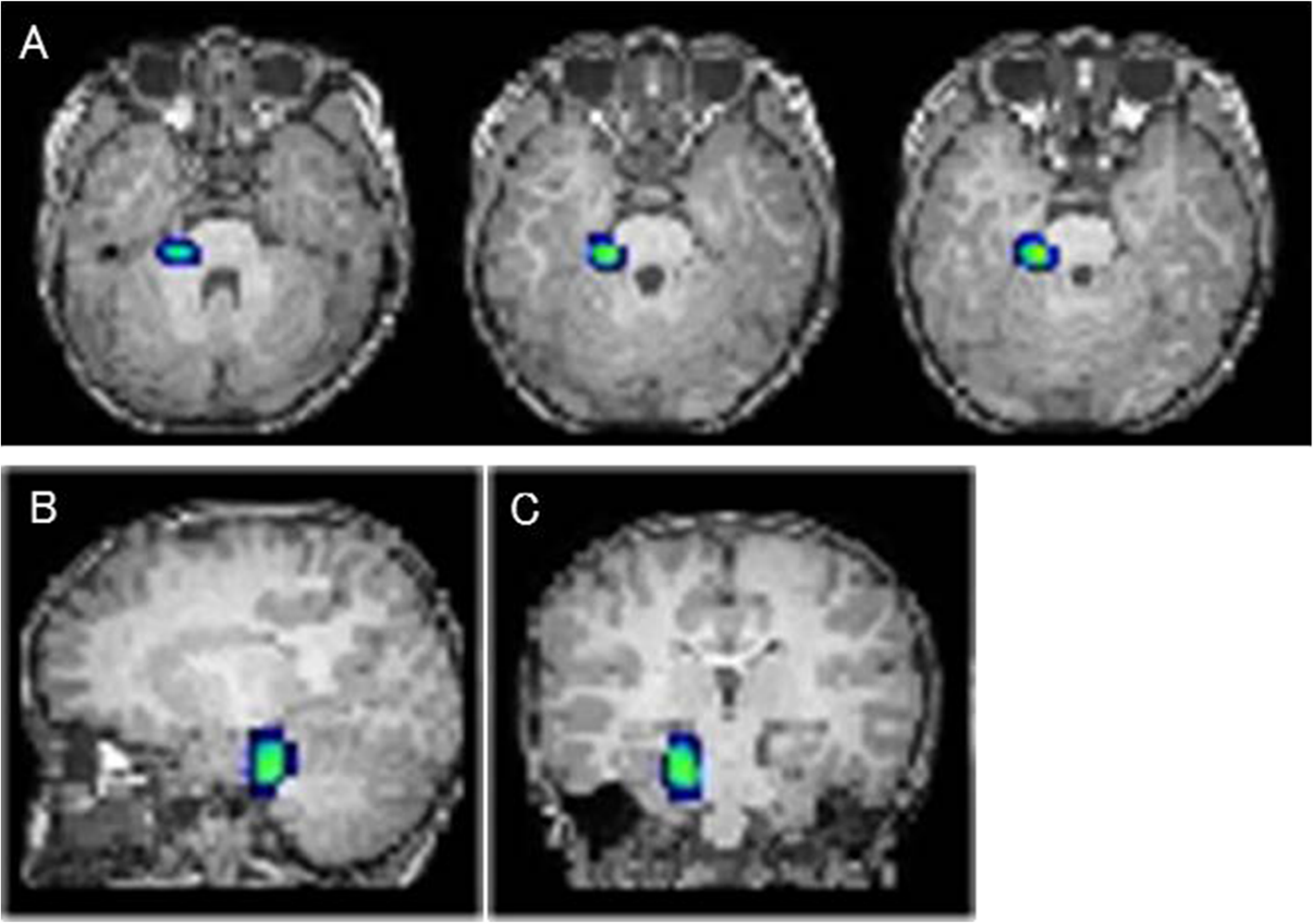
SPECT co-registered to MRI (SISCOM) analysis in Patient 2. A) Transverse, B) sagittal, and C) coronal images. A focal increase in perfusion is noted at the right trigeminal root entry zone during a right-sided attack.
